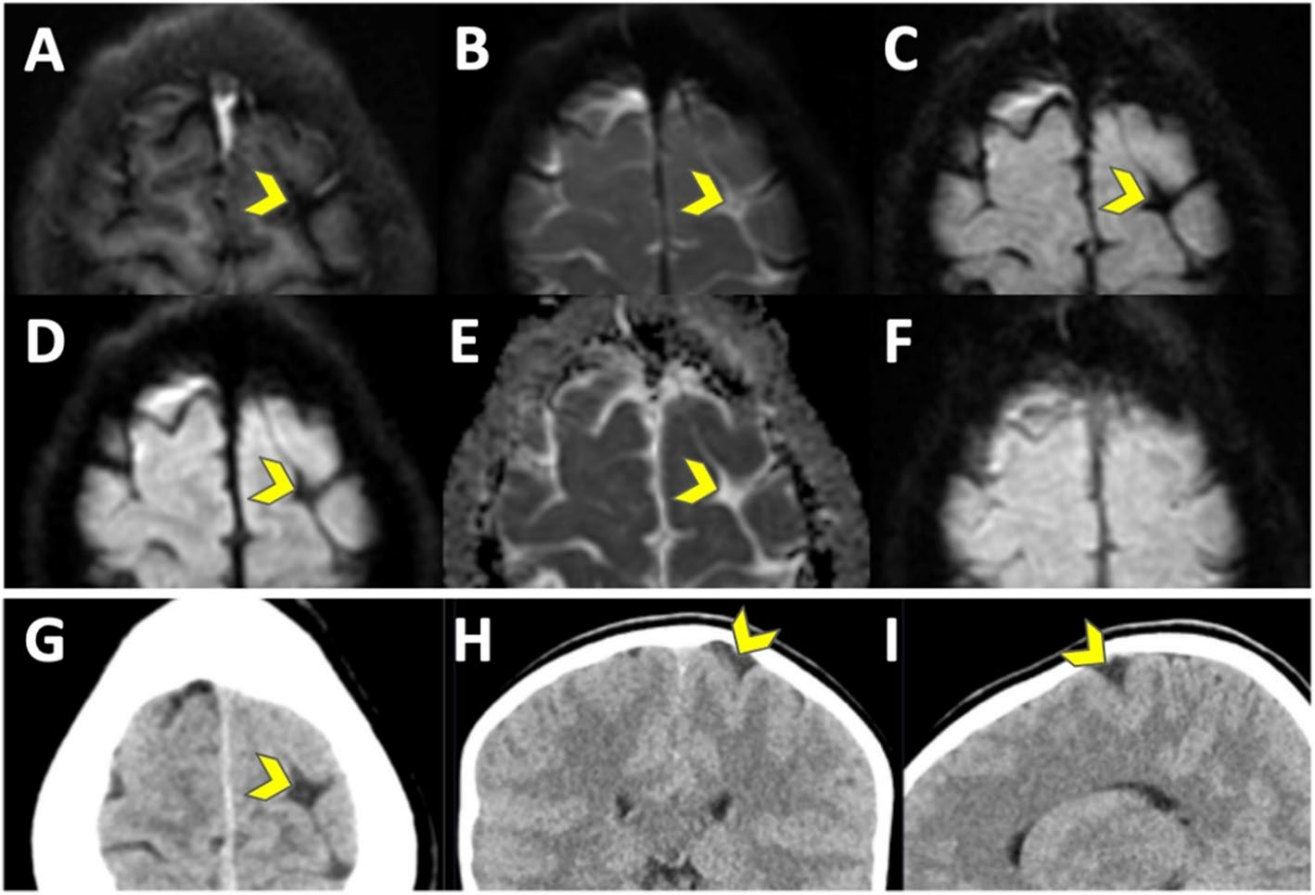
ID11. A wide sulcus (yellow arrowhead) reported together with suspected underlying focal cortical dysplasia, the latter not confirmed at the side-by-side consensus. T1-FLAIR (A), T2 (B), T2-FLAIR (C), DWI (D), ADC (E), T2* (F) EPIMIX images, and axial (G), coronal (H), sagittal (I) soft tissue window CT images
